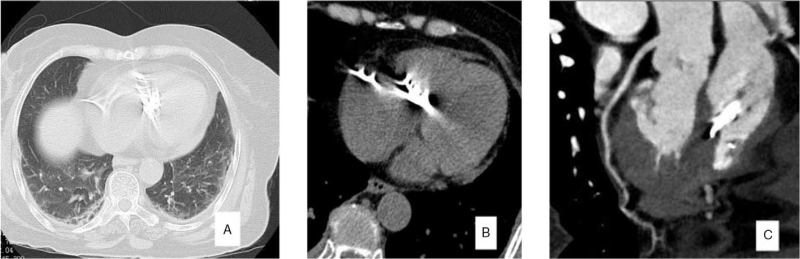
CT and coronary angiography of the chest showed multiple compact and strip-shaped images in the heart.
